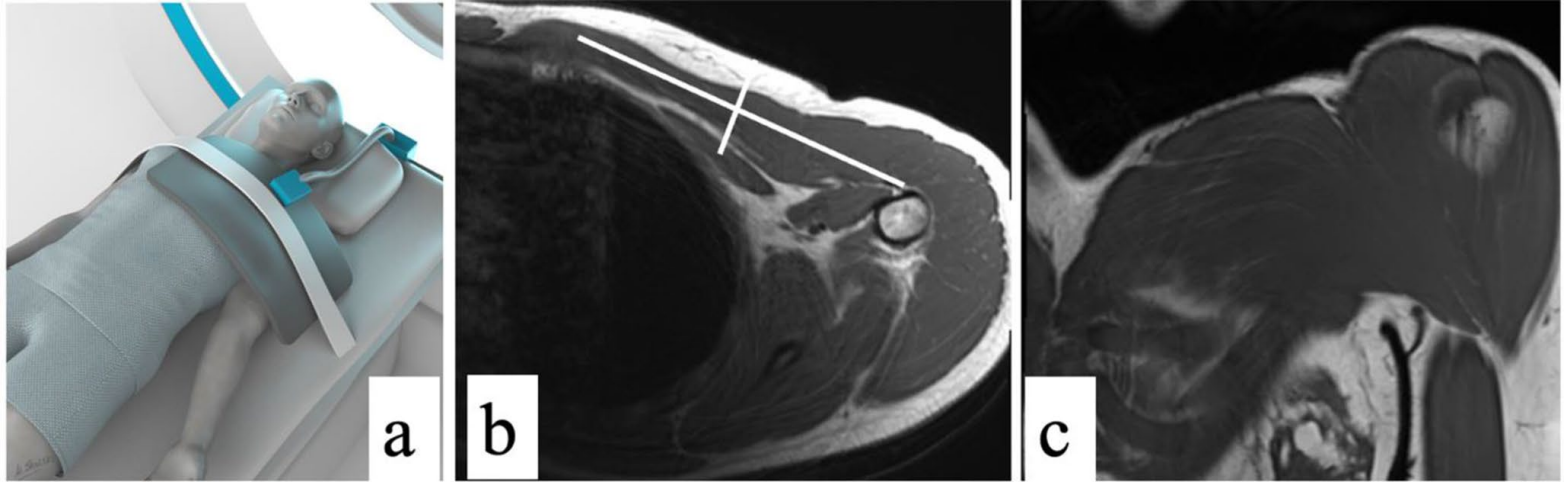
Sample supine patient positioning for MRI assessment of the pectoralis major is shown (a). In this example, the patient is positioned supine with a flexible coil placed over the chest on the side of interest strapped tightly to the patient to minimize respiratory artifact, and the arm is slightly abducted with external rotation to improve visibility of the tendon. The MRI field of view on a selected axial T1-weighted (b) and oblique coronal T1-weighted (c) image are shown. Reference lines for the coronal and sagittal plans are shown in (b). The coronal section is set parallel to the majority of the fibers of the pectoralis major muscle. The sagittal section should be set perpendicular to the coronal section
